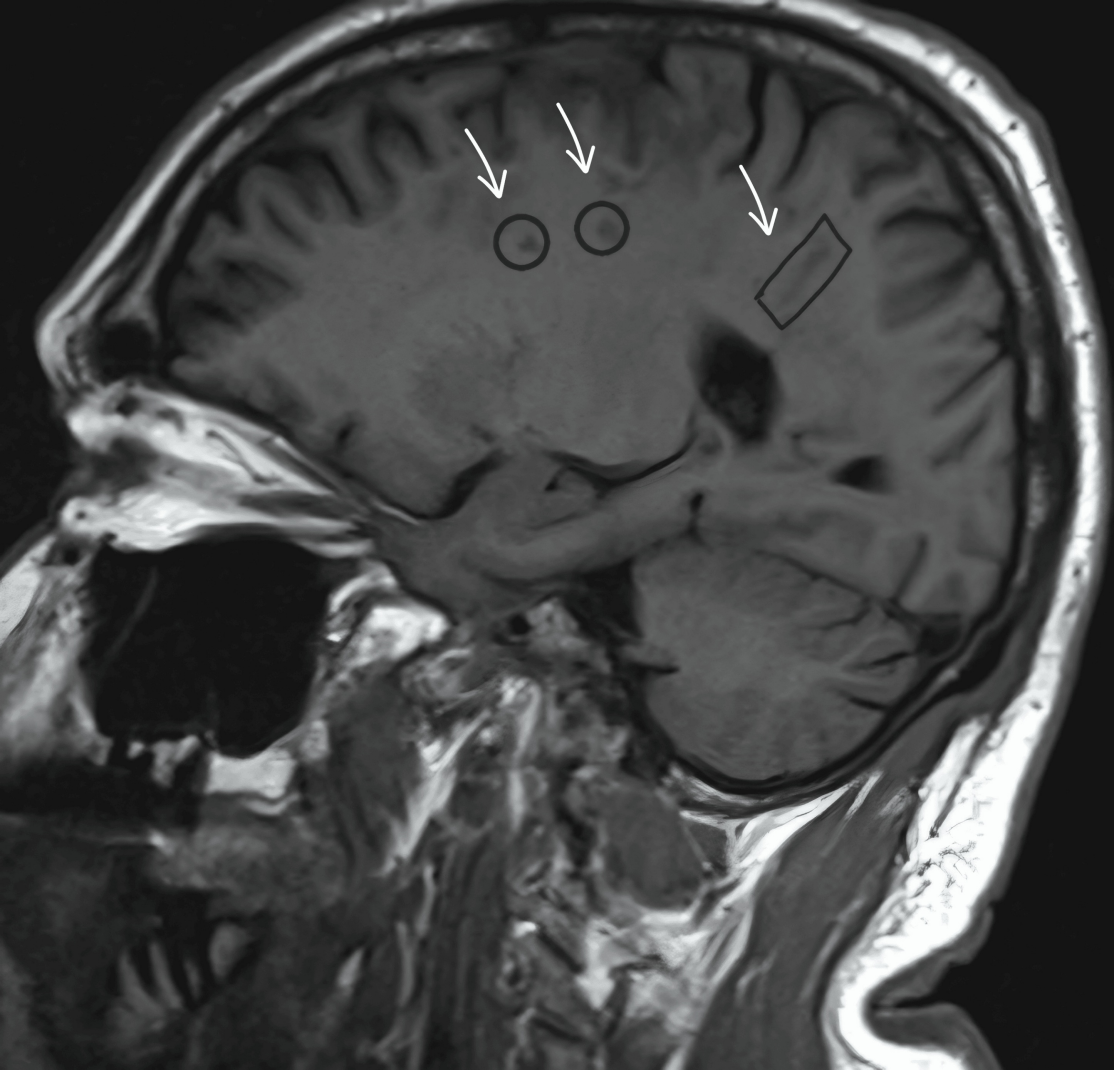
MRI brain - air pockets encircled and marked by white arrows MRI: magnetic resonance imaging
